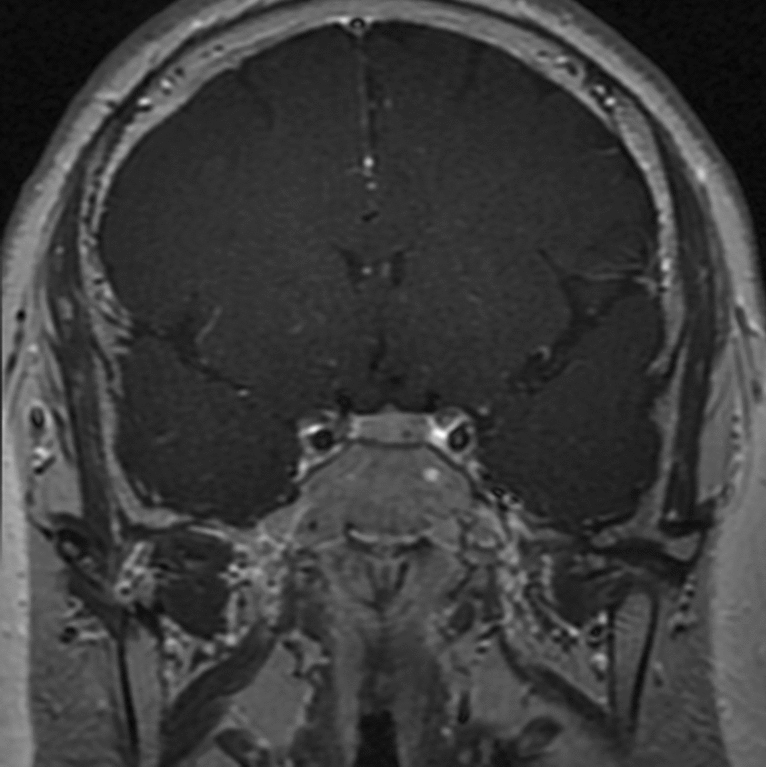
Pituitary dynamic enhanced MRI for pituitary adenomas. A 3 mm pituitary adenoma was shown in the left sella with delayed enhancement.
